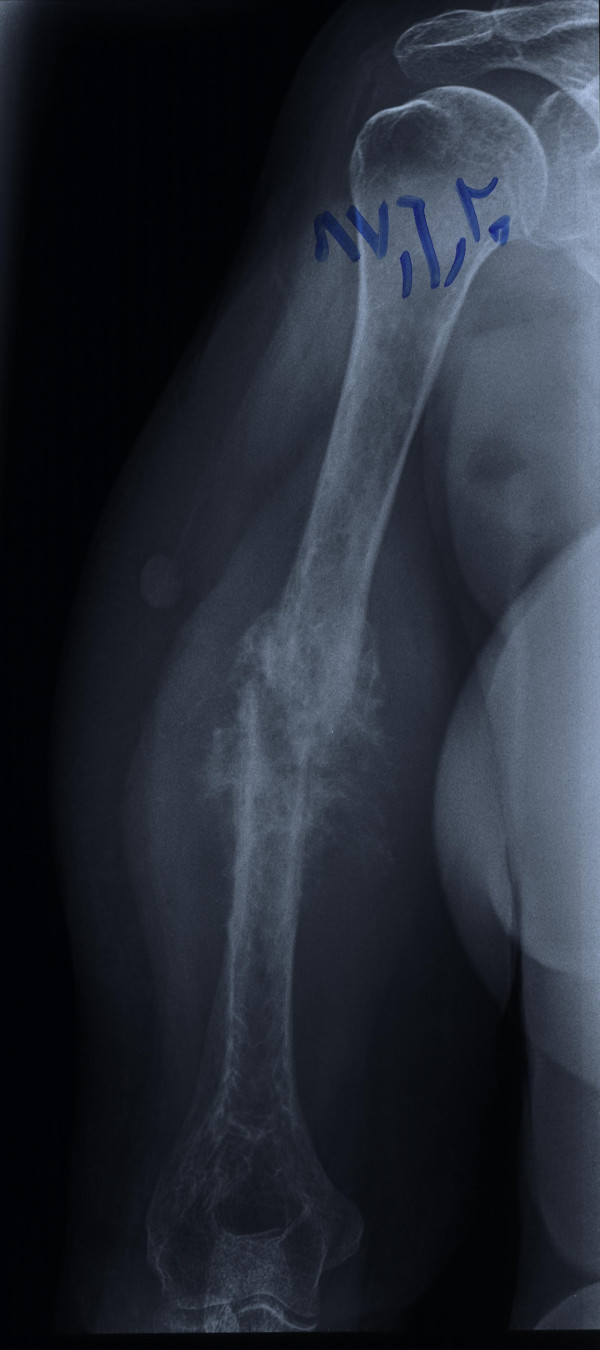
Plain radiograph showing a tumor characterized by a fracture in the mid-shaft of the right humerus with a large soft tissue mass.
